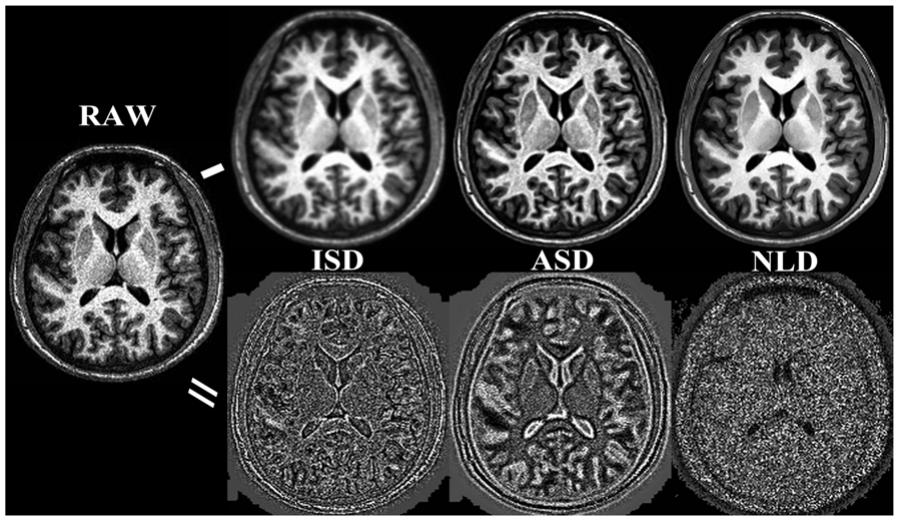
The effect of PDE-derived smoothers on an Individual Degraded Structural Image. PDE-based MRI denoising. An individual T1 structural image (RAW) were denoised by three PDE-based smoothing kernels: ISD, ASD and NLD. The first row shows the denoised T1 brain images as well as the second row depicts the difference images between each of the three smoothers and the raw noisy image.
